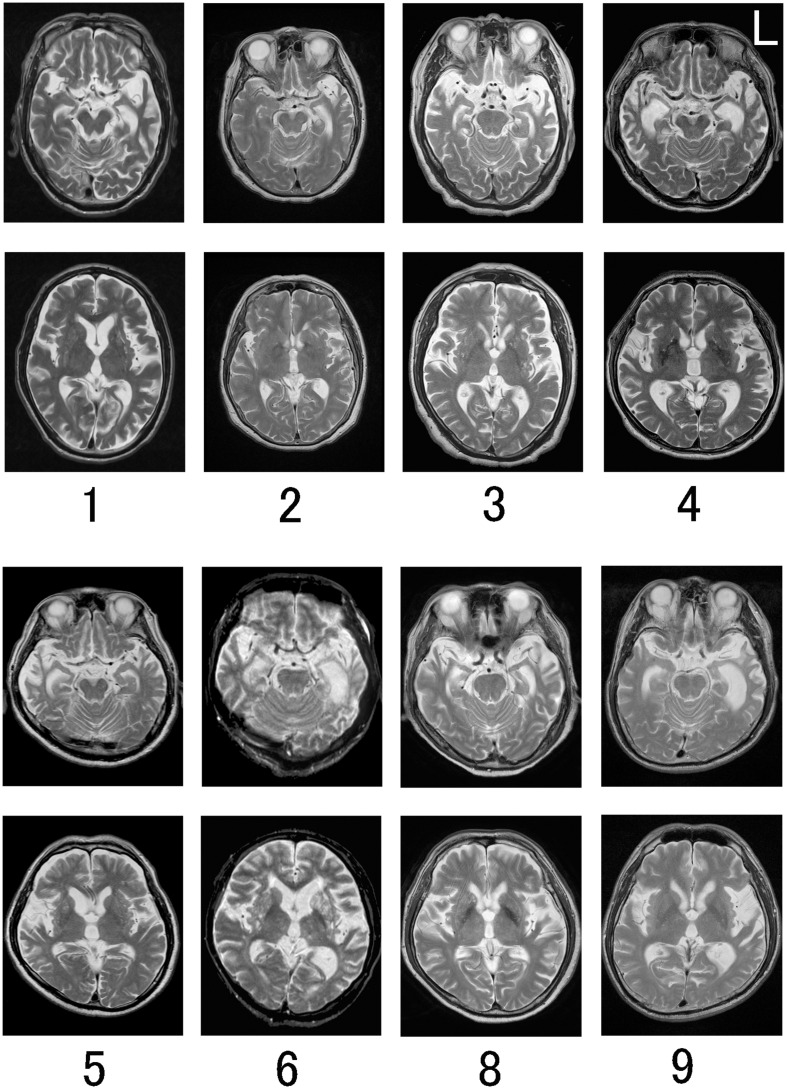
MRI T2-weighted images of eight semantic dementia patients. Number corresponds to the patient number in Table 1. MRI of patient 7 is not shown. Temporal lobe atrophy was left-sided only (Patients 1 and 2), left-right nearly equal (Patients 3, 4, and 8), right-predominant (Patient 5), and left-predominant (Patients 6 and 9).
